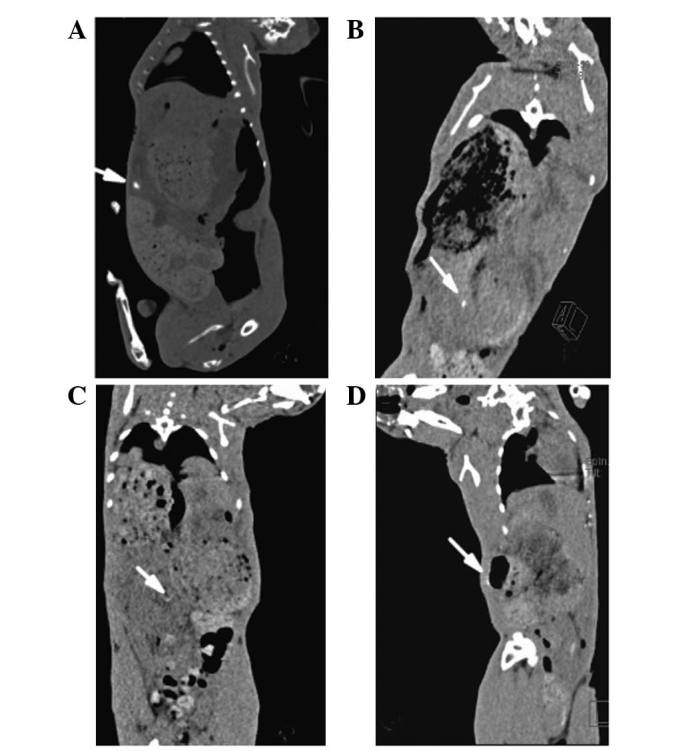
Computed tomography scan on (A) the day of surgery and (B) the 1st, (C) 2nd and (D) 3rd week post-implantation. The stents degraded gradually.
